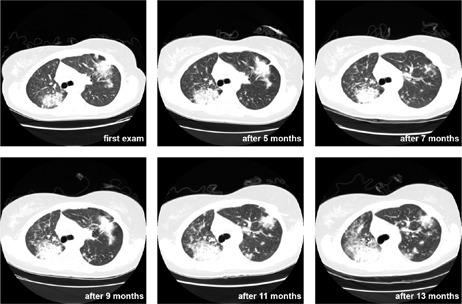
Images near carina level from CT exams of patient I, acquired in six different moments: at first exam (when the tumor was found) and other five exams acquired at 5, 7, 9, 11 and 13 months after the first exam.
